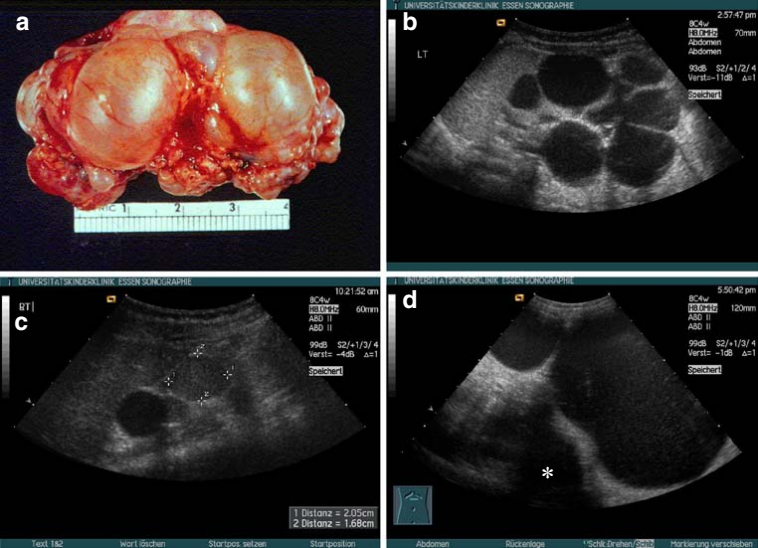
Multicystic dysplasia: a macroscopic appearance; b typical example of sonographic appearance; c multicystic kidney with nephroblastoma (*marked with calipers*); d giant-size multicystic kidney (approximately 900-ml volume) in a 2-year-old girl, crossing the midline (transverse section, * vertebral column)
